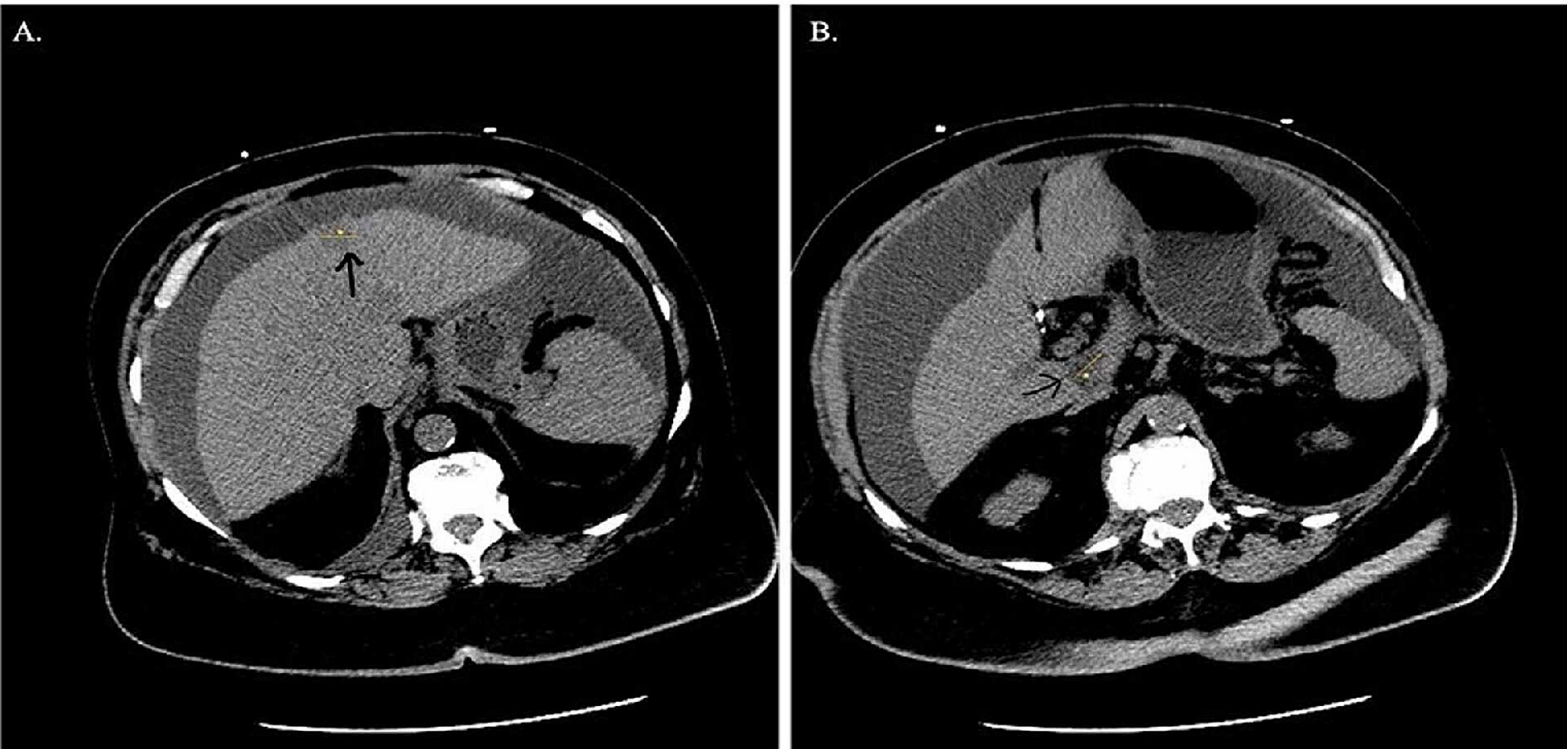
Abdominal CT scan showing hepatic (A) 17.5 mm hypodense segment 4 lesion and (B) 21 mm hypodense segment 1 lesion
